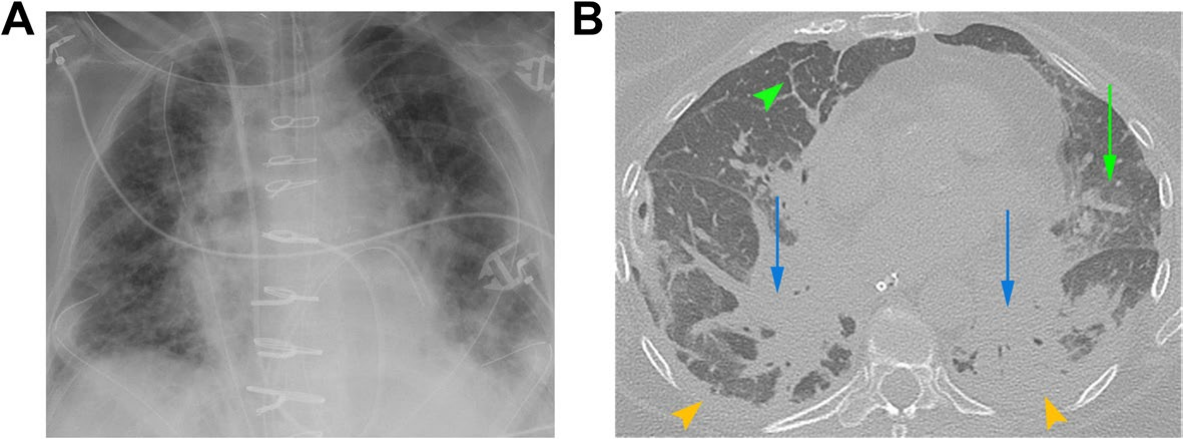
PGD in a 62-year-old female with bilateral lung transplants. A Frontal radiograph obtained on postoperative day 2 after worsening respiratory status including respiratory alkalosis and increased FiO2 requirements shows bilateral hazy opacities and interstitial markings consistent with pulmonary edema, increased from the baseline radiograph obtained on day 1 (not shown). B Axial CT (lung window) obtained postoperative day 5 after slow weaning from mechanical ventilation, shows interlobular septal thickening (green arrowhead), groundglass opacity (green arrow), bibasilar atelectasis (blue arrows), and pleural effusions (yellow arrowheads), representing typical HRCT findings of pulmonary edema. Diagnosis of grade 3 PGD was made based on a PaO2/FiO2 ratio of < 200 and the radiologic findings
